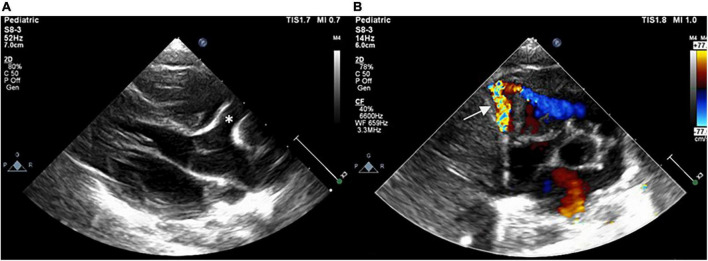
Postnatal echocardiogram showing dilated RCA (asterisk) with a fistula (arrow) drained into the RV in (A) parasternal long-axis view and (B) short-axis view. RCA, right coronary artery; RV, right ventricle.
